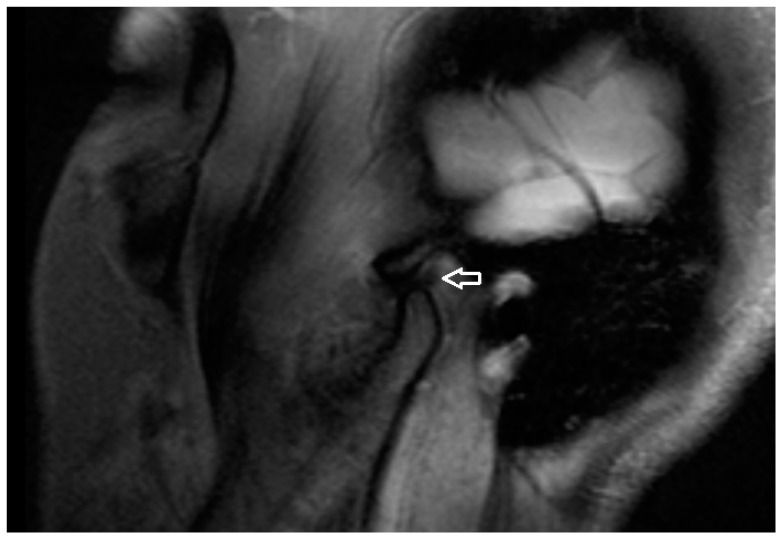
PD TSE SPAIR Sagittal MRI scan (articular disc—white arrow).
